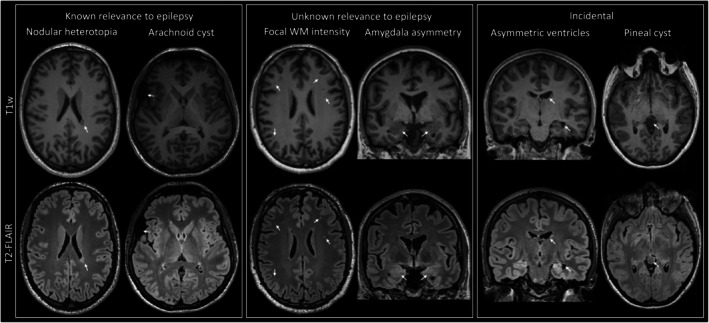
Exemplar MRI‐positive cases across the three lesional categories. Known relevance to epilepsy: Nodular heterotopia of the left supra‐trigonal periventricular region (left) and arachnoid cyst overlying anterior aspect of the right sylvian fissure, abutting the inferior frontal gyrus superiorly and the temporal pole inferiorly (right). Unknown relevance to epilepsy: Multiple focal white matter hypointensities (T1w) and hyperintensities (T2‐FLAIR) (left) and amygdala asymmetry (right; note additional ventricular asymmetry). Incidental: Asymmetric lateral ventricles, including frontal and temporal horns (left) and pineal cyst (right).
